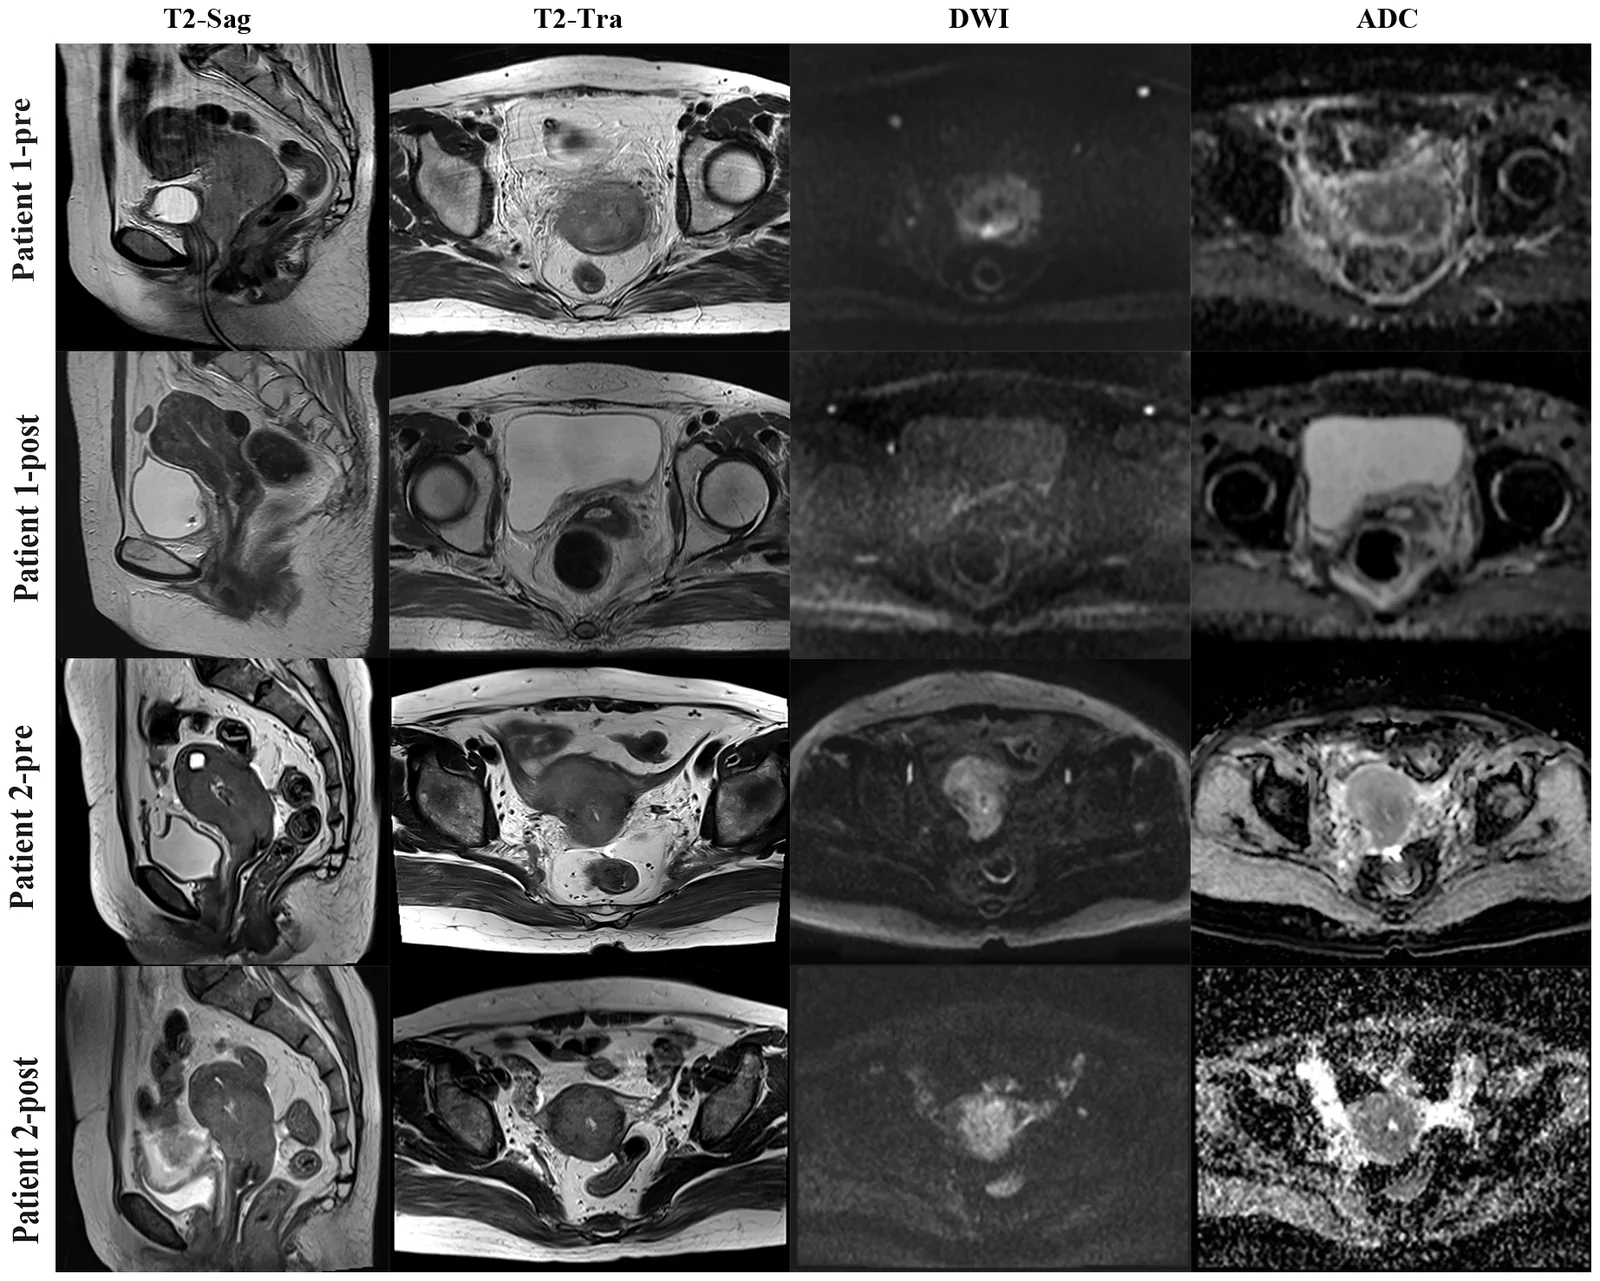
Pelvic MRI examples of chemotherapy responders and non-responders before and after preoperative neoadjuvant chemotherapy.
